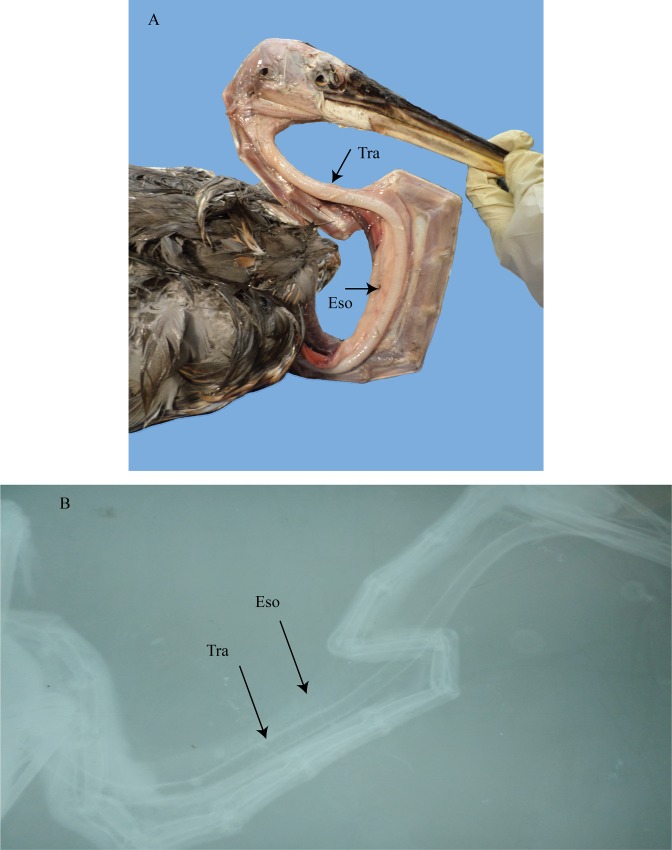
Ardea herodias*. (A) dissection. (B) radiograph. Tra = trachea; Eso = esophagus.
